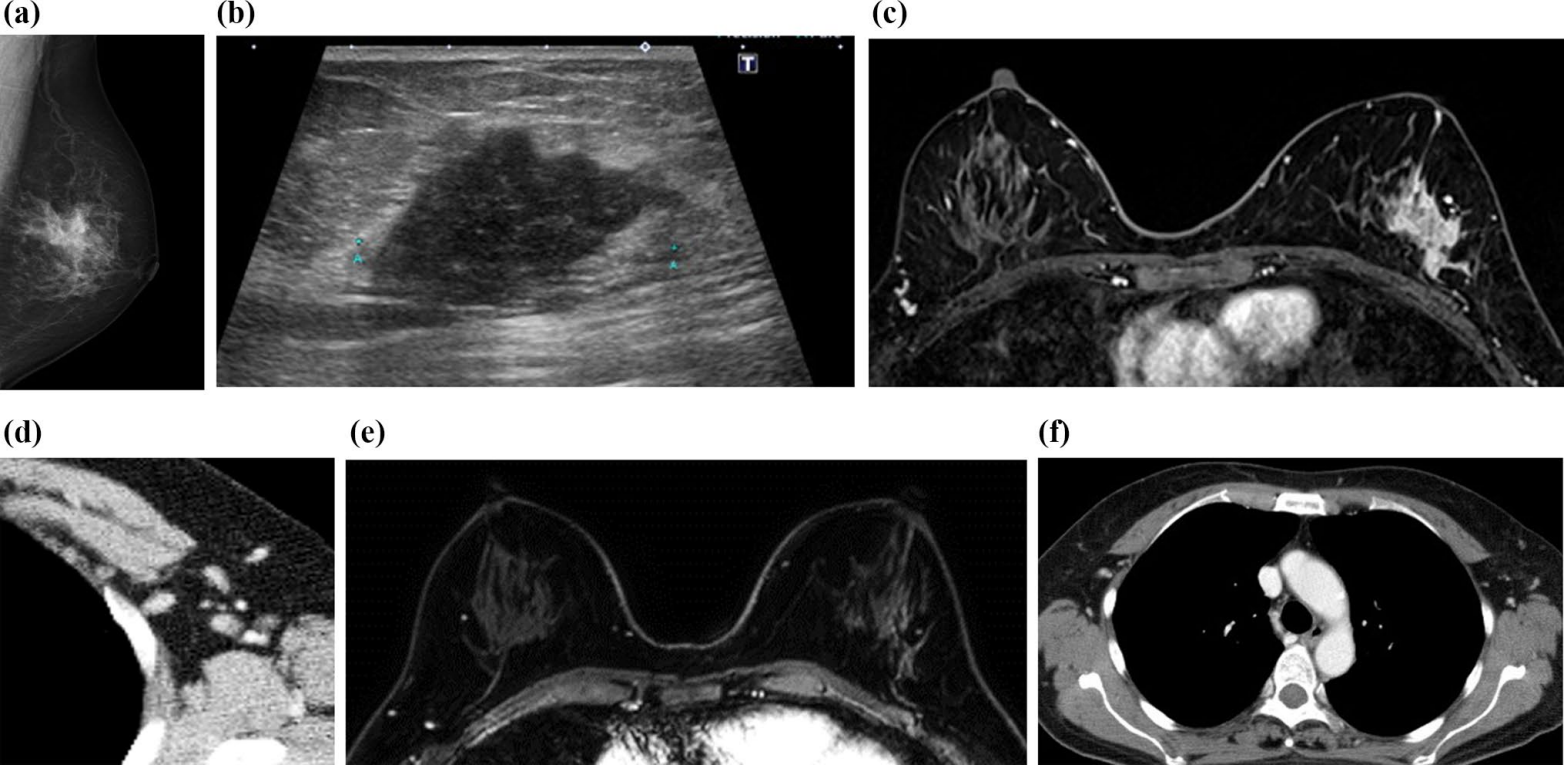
Pre- and post-treatment imaging of a woman in her 50s with HER2-positive breast cancer (Case 2). a–d Pretreatment imaging: a Mammography of the left breast, b Ultrasonography of the left breast, c Initial phase of dynamic DCE MRI, d Contrast-enhanced CT. Pretreatment imaging revealed a 4-cm tumor in the left breast with axillary lymph node involvement (a–d). e–f Posttreatment imaging following trastuzumab and pertuzumab in combination with chemotherapy: e Initial phase of dynamic contrast-enhanced (DCE) MRI, f Contrast-enhanced CT. Posttreatment MRI demonstrated no residual invasive carcinoma and no evidence of residual lymphadenopathy (e, f). The patient achieved pCR, validated by pathology, which correlated with the complete response seen on imaging
